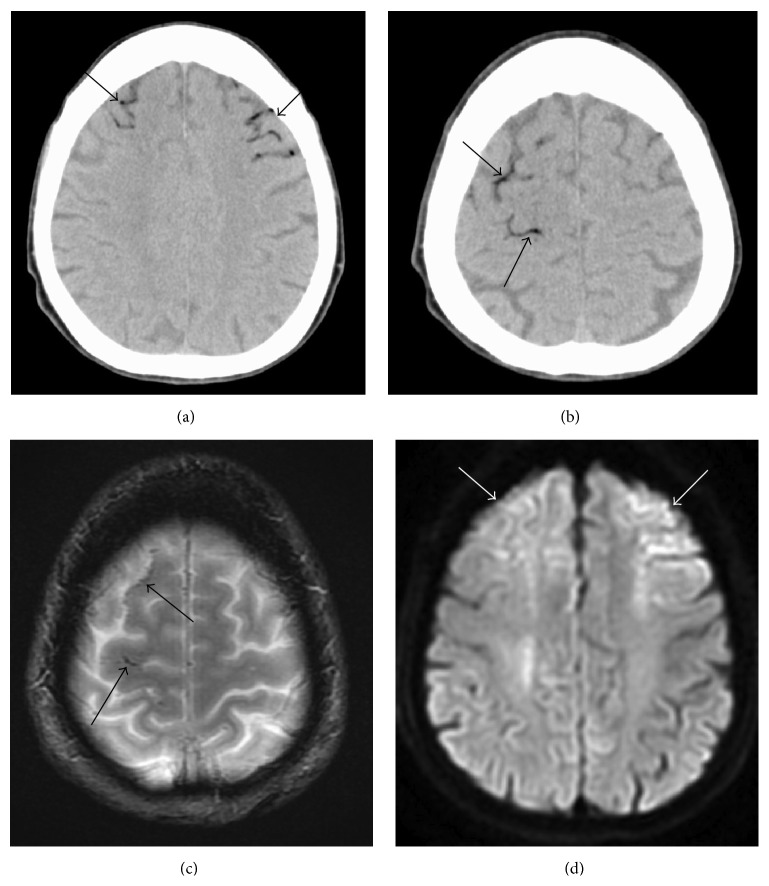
A 70-year-old woman (case 1). (a, b) Brain CT performed immediately after her arrival at our hospital (60 min after the estimated CAE onset) shows air inflow in cortical veins in the bilateral frontal areas and the right parietal area reflecting buoyancy of the air (arrows). (c) T2^*∗*^WI obtained 30 minutes after the CT scan at the same level as in (b). There was less air than in (b) (arrows). (d) DWI obtained 30 min after the CT scan showing cortical areas with restricted diffusion near the air (arrows).
